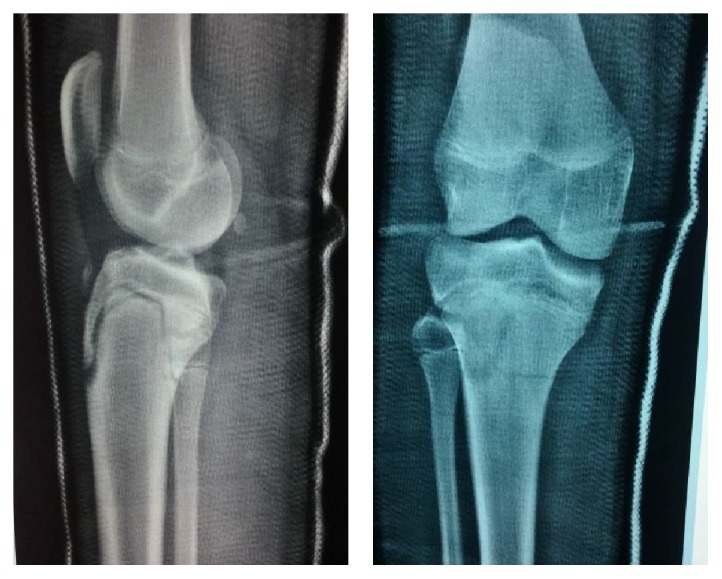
Plain radiographs one week after reduction.
